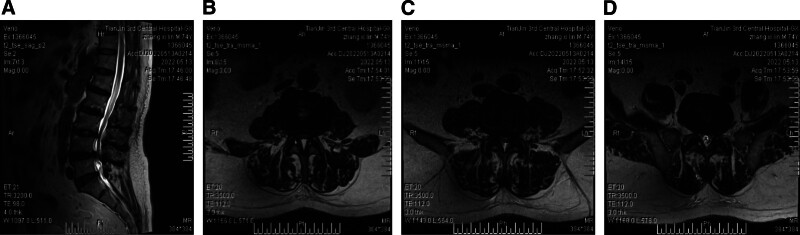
Preoperative lumbar spine MRI findings. (A) Sagittal plane showing lumbar disc herniation at L3/4, L4/5, and L5/S1 levels. (B) Axial plane at L3/4 level demonstrating thickening of the ligamentum flavum and spinal canal stenosis. (C) Axial plane at L4/5 level demonstrating thickening of the ligamentum flavum and spinal canal stenosis. (D) Axial plane at L5/S1 level demonstrating thickening of the ligamentum flavum and spinal canal stenosis. MRI = magnetic resonance imaging.
